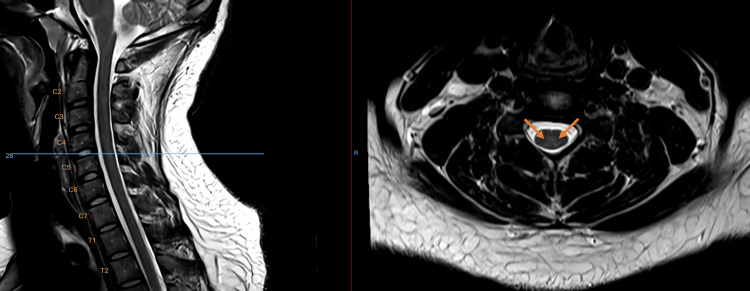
Axial T2-weighted MRI (3T) imaging of the cervical spine (C4-5)
